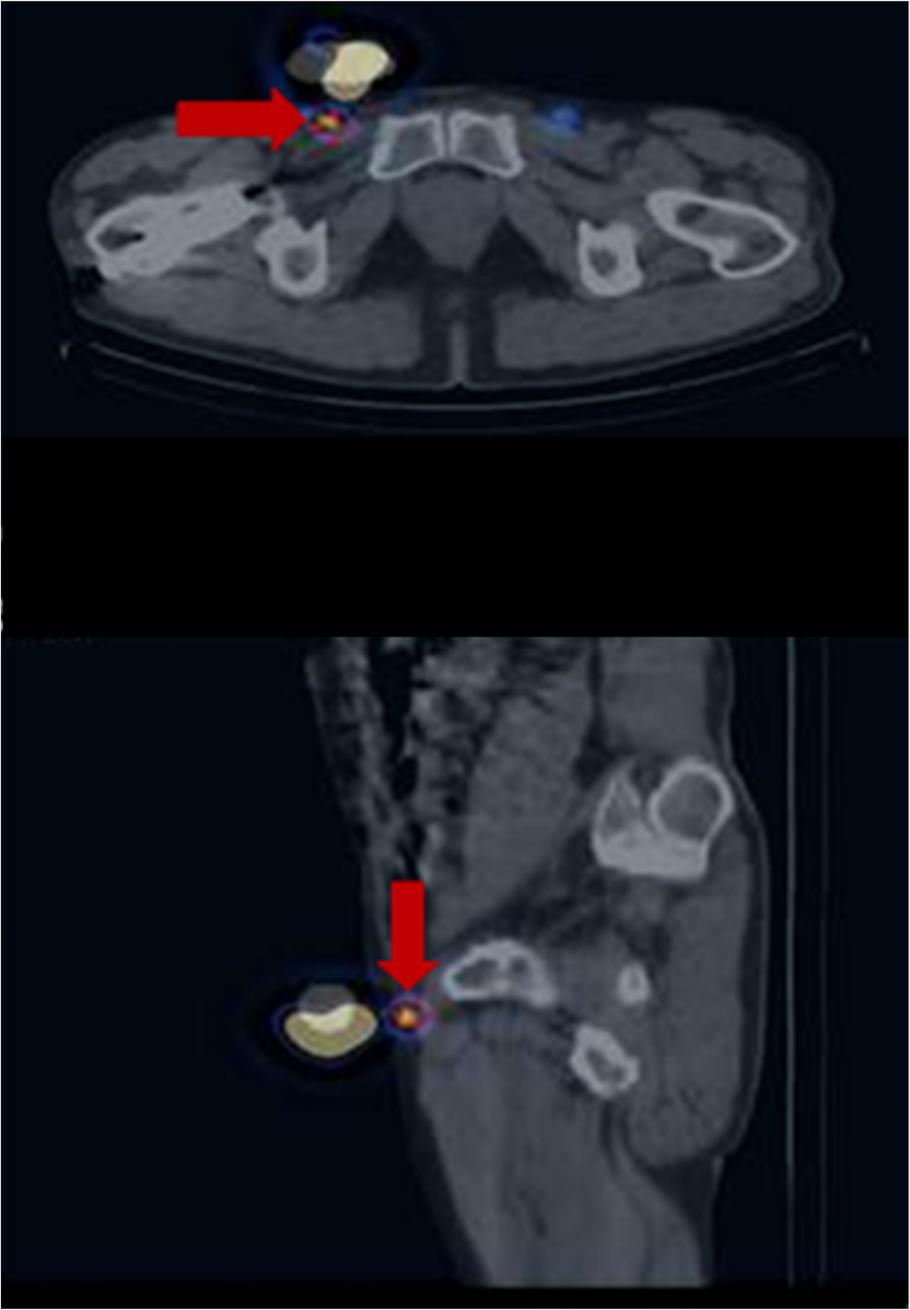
SPECT/CT clearly localizes a node (*red arrows*) that was missed on planar imaging due to adjacent intense activity
